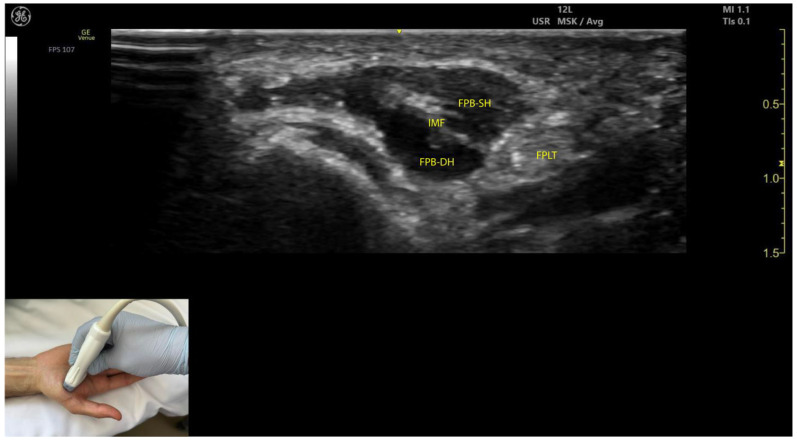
Ultrasound anatomy of the flexor pollicis brevis (FPB) with key landmarks: FPB-SH—flexor pollicis brevis superficial head; FPB-DH—flexor pollicis brevis deep head; IMF—intramuscular fascia; FPLT—flexor pollicis longus tendon.
